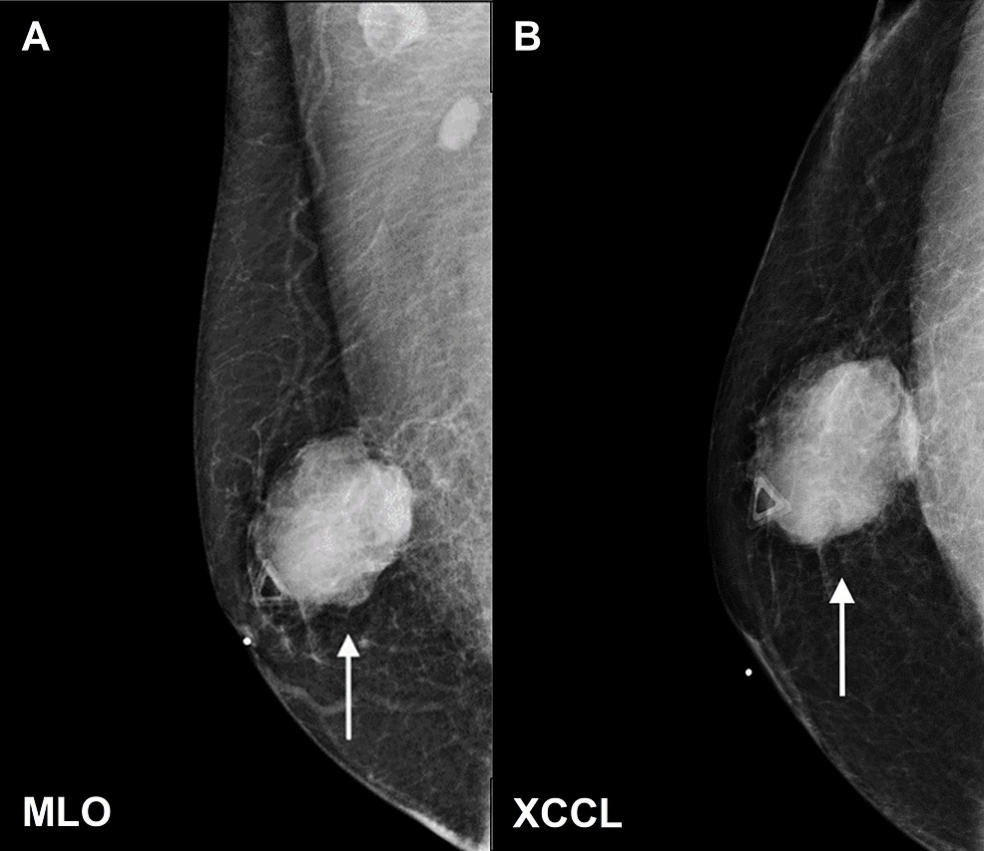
Mammography of the right breast demonstrates a high density and irregular mass with microlobulated margins located at nine o’clock, 3 cm from the nipple. (A) The mediolateral oblique (MLO) and (B) exaggerated craniocaudal view (XCCL) are presented. Two normal axillary lymph nodes are also observed in MLO view that were determined to be benign upon further evaluation with ultrasound and measurement of metabolic activity.
